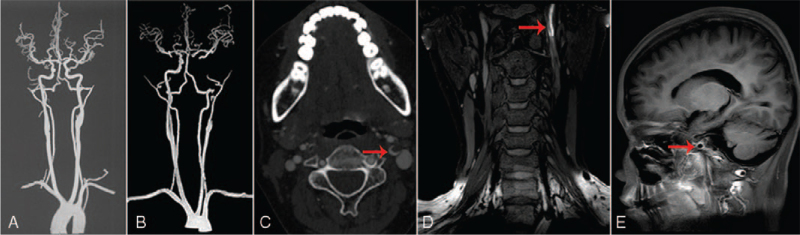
CT angiography 9 months before admitted showed normal extracranial and intracranial vessels (A). CT angiography after admitted revealed occlusion in left internal carotid artery and a crescentic shape of real lumen (B and C). Carotid plaque high-resolution MRI showed annular enhancement of vascular wall (D and E). MRI = magnetic resonance imaging.
